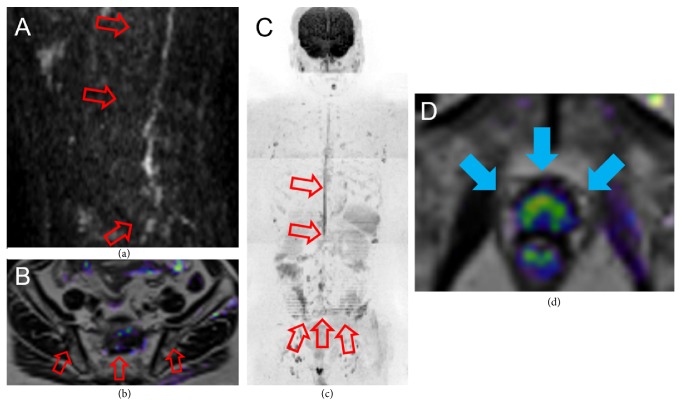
WB-MRI at the time of CRPC diagnosis. Sagittal DWI of the spine (a), coronal DWI + T2 fusion (b), and whole-body DWI (c) showed few high signals. Coronal DWI + T2 fusion of the pelvis (d) revealed diffuse high signal in the prostate. WB-MRI, whole-body magnetic resonance imaging; CRPC, castration-resistant prostate cancer; DWI, diffusion-weighted imaging.
